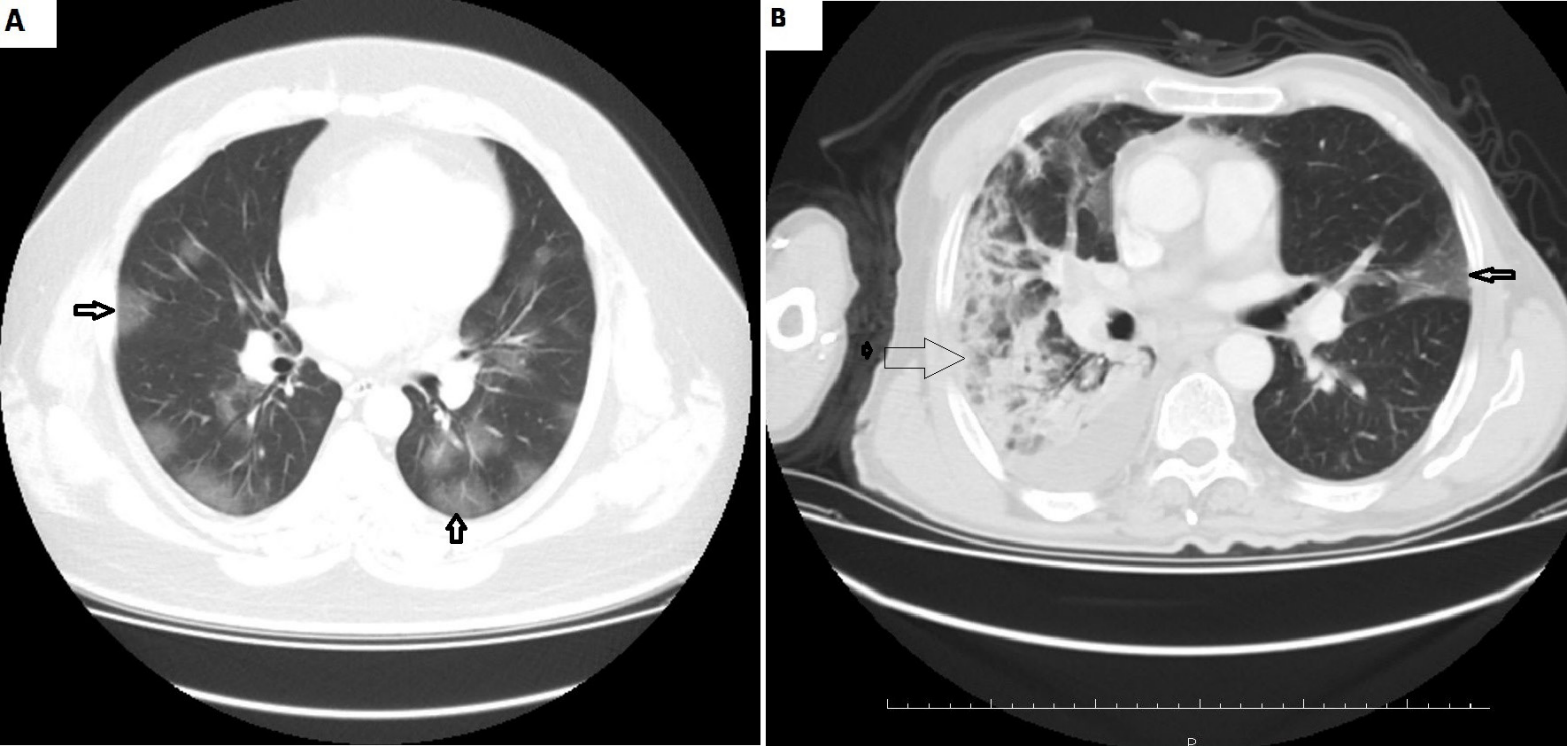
The presence of GG0 (A) and GGO together with widespread consolidation (B) in the axial lung window section of admission chest CT of these patients (1a: 64 years age, male, with hpertension; 1b: 43 years age, male) who lost their lives, draw attention. Thick little arrows: ground glass opacity; thin big arrow: consolidation.
